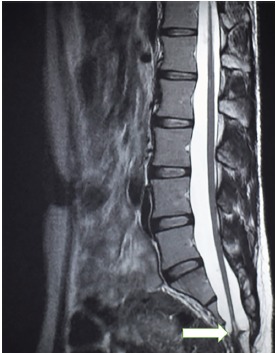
Sagittal view of a T2-weighted magnetic resonance image of the lumbar spine showing the conus medullaris of the tethered cord reaching to inferior edge of the second sacral vertebrae (arrow).
